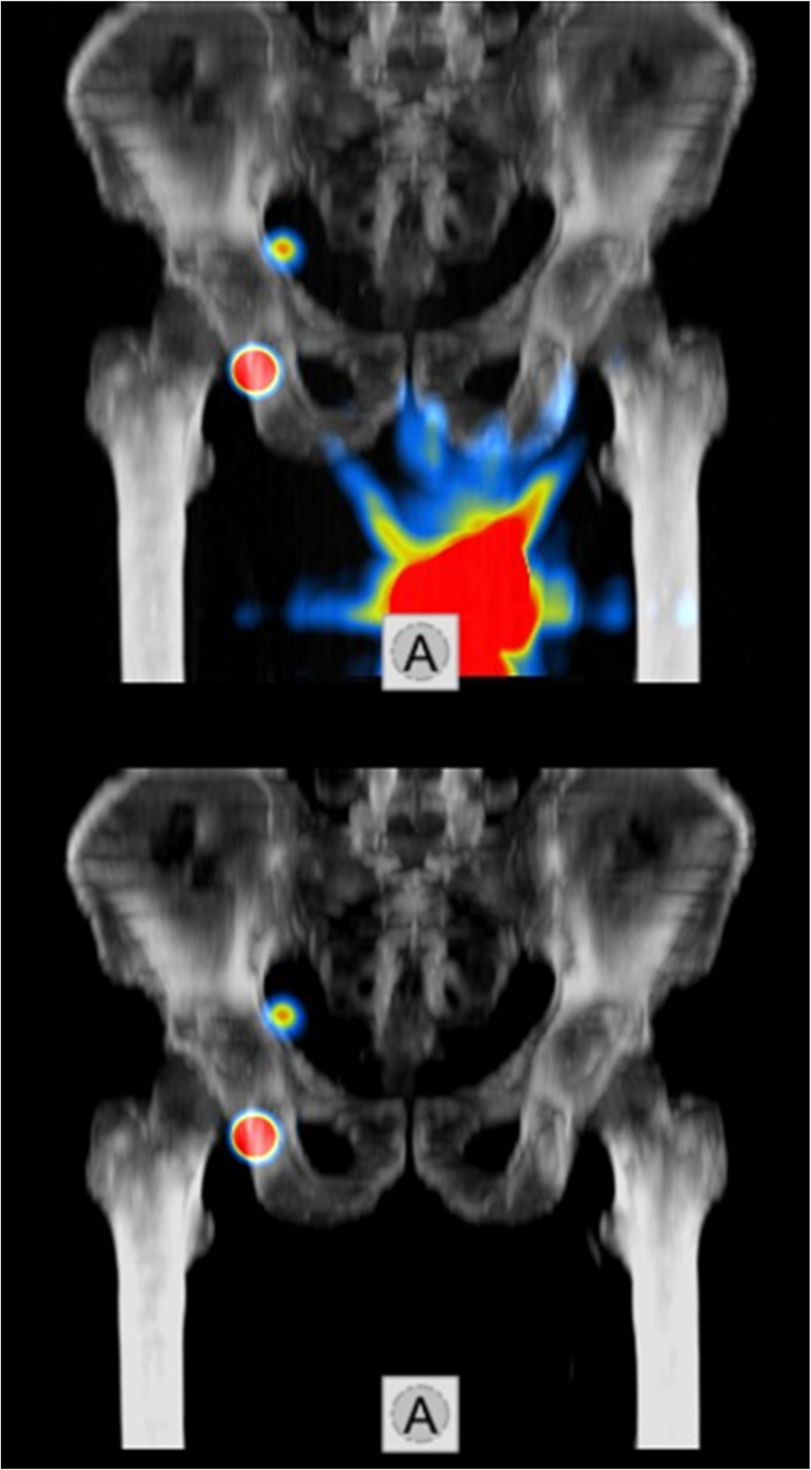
Preoperative 3D-MIP-Fusion imaging of pelvic SPECT/CT in a patient prior to application of the program (*above*) and after application of the method with elimination of the tumor region/application site (*below*) as well unchanged visualization of the SLN (hot spots) in the right inguinal region
